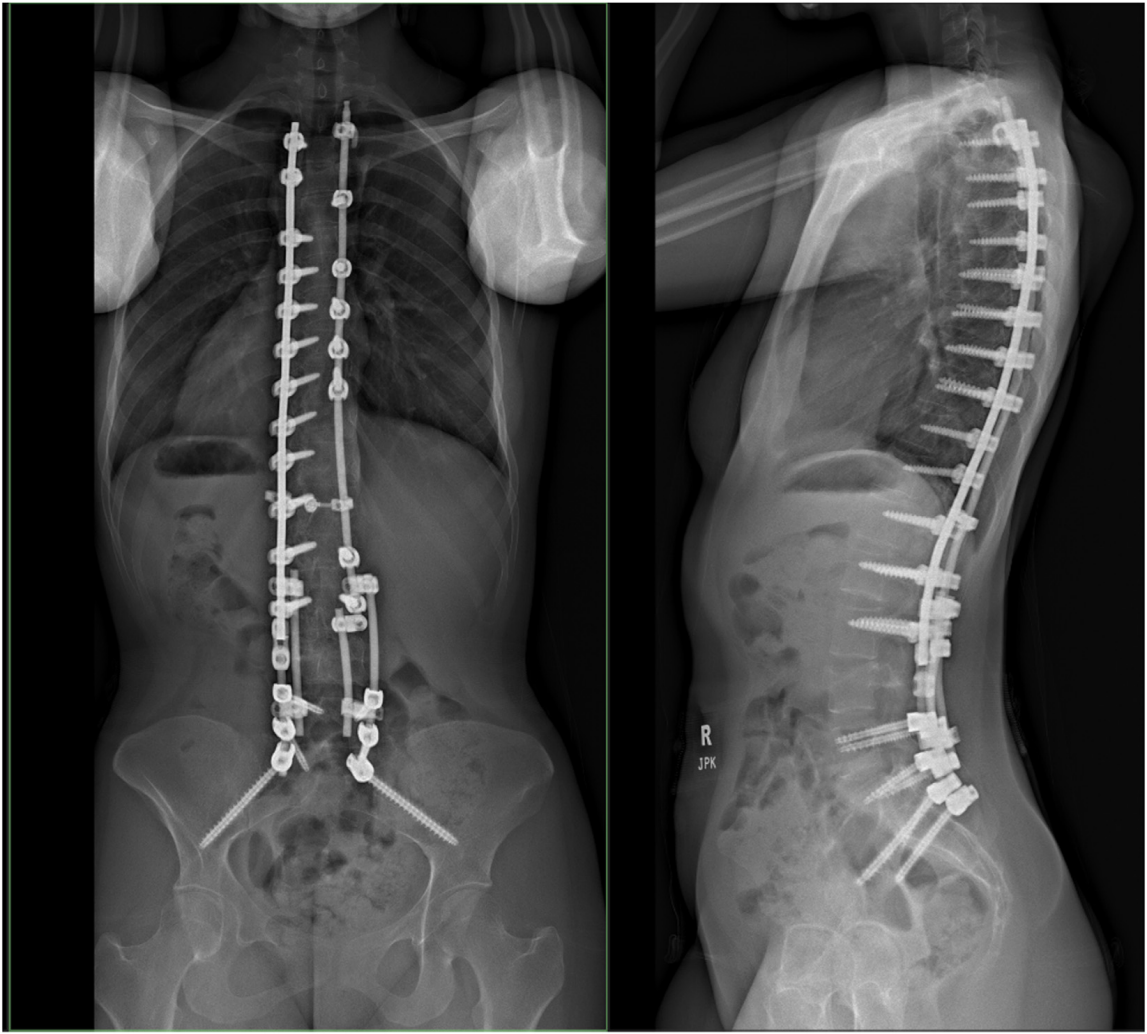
Anteroposterior and lateral plain radiograph after management of the L4 burst fracture.
